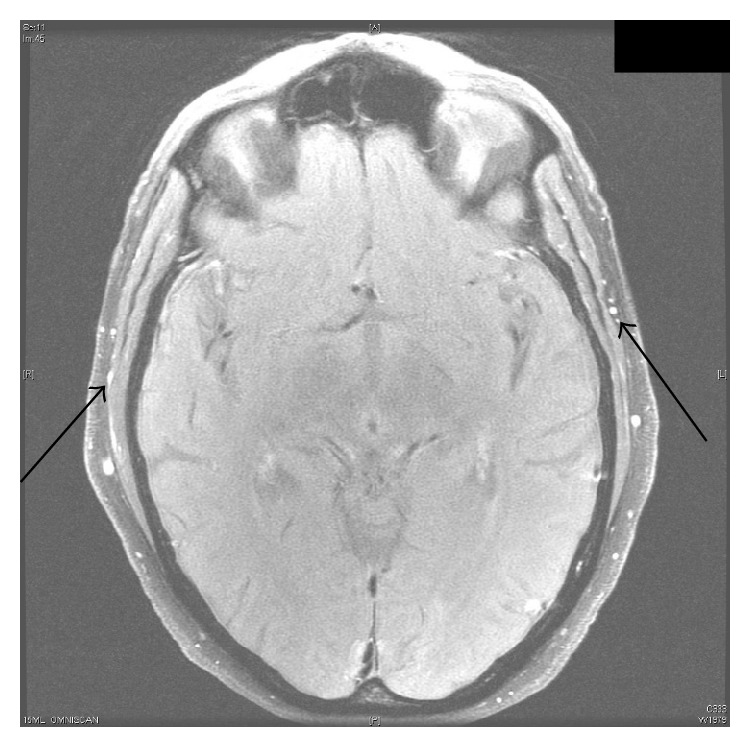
MRI showing mural thickening and enhancement of temporal artery “branches” bilaterally (arrows).
